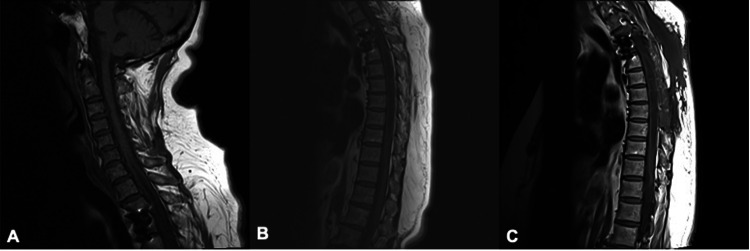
Preoperative and postoperative MRI imaging. (A) Preoperative T1 sequence of the cervical spine with hyperintensity posterior to the cord beginning at C7/T1. Syringomyelia may also be visualized. (B) Preoperative T1 sequence of the thoracic spine demonstrating hyperintensity posterior to the cord in the thoracic spine. Syringomyelia is also visualized. (C) Three-month post-operative T1 sequence of thoracic spine demonstrating decreased hyperintensity along the posterior cord relative to preoperative imaging. Syringomyelia may still be visualized.
